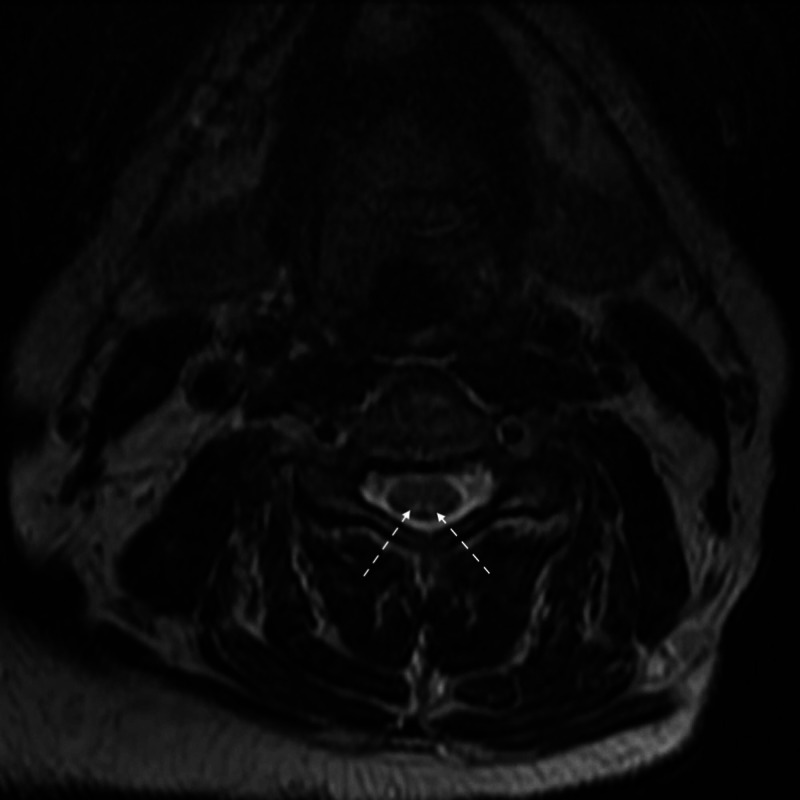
T2 axial MRI of the cervical spine showing increased signal attenuation of the dorsal columns (white arrows) MRI, magnetic resonance imaging.
